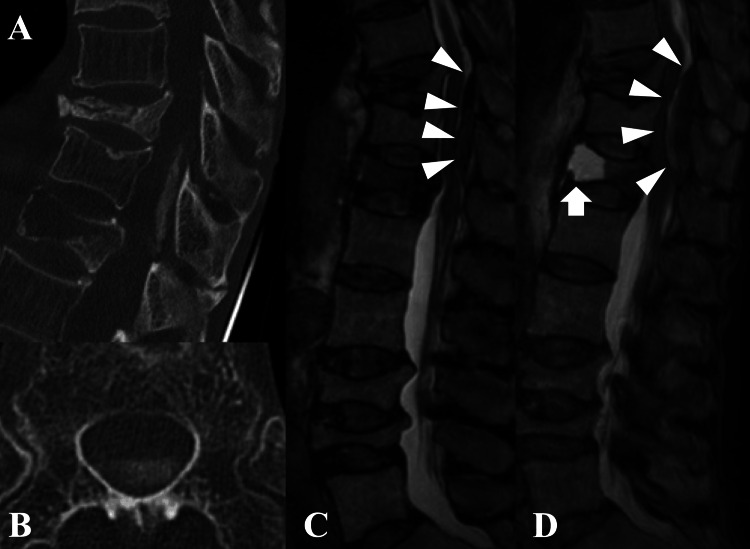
Thoracic CT and MRI A: Sagittal CT showing a space-occupying lesion on the dorsal epidural surface of T12. B: Axial CT showing a space-occupying lesion on the dorsal epidural surface of T12. C: Sagittal T2-weighted MRI revealed an epidural lesion (arrowheads). D: Sagittal T2-weighted MRI when paralysis and sensory disturbance worsened. The image confirmed the enlarging epidural lesion (arrowheads) and demonstrated niveau formation within the T12 vertebral body (arrow).
